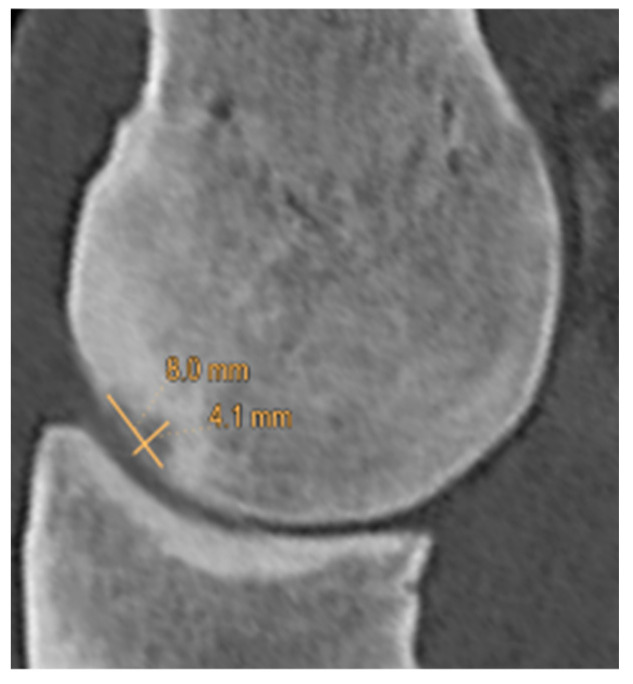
Sagittal computed tomographic reconstruction of the sagittal ridge of the third metacarpal bone (dorsal is to the left) showing measurements of the proximodistal (8.0 mm) and dorsopalmar (4.1 mm) extents of a hypoattenuating lesion extending towards trabecular bone. Note the hyperattenuating area surrounding the lesion.
